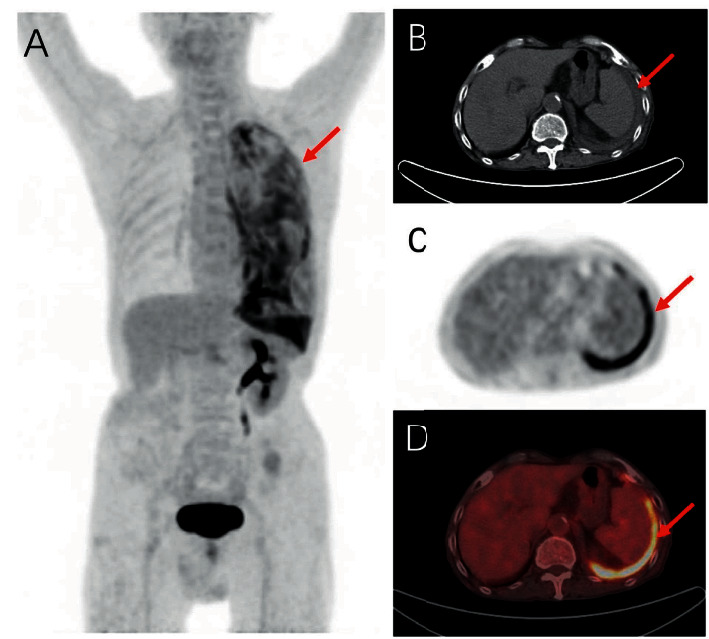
An 85-year-old man presented with cough and chest tightness and was diagnosed with TBP by thoracoscopic biopsy. (a, c, d) Diffused 18F-FDG uptake of the left pleura (red arrow). (b) Lamellar thickening of the left pleura (red arrow).
